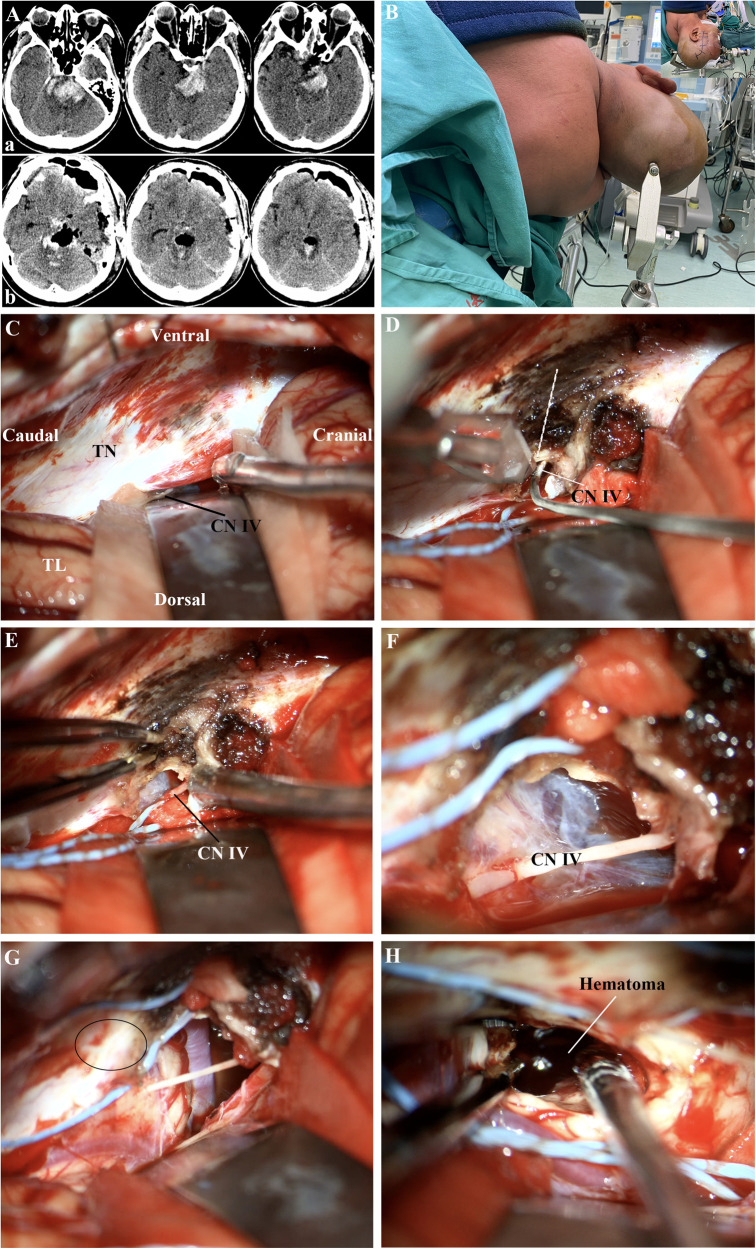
Left subtemporal trans-tentorial approach for pontine hematoma evacuation. (A) Cranial CT scans reveal pontine hemorrhage (a) and postoperative follow-up imaging (b) (B) The patient was placed in the left lateral position with the zygomatic arch maintained horizontal; the head was flexed downward at approximately 20°, and a preauricular curved incision was made (Upper right inset). (C) The arachnoid membrane at the temporal base was dissected to release cerebrospinal fluid, and gravity-assisted retraction of the temporal lobe was performed to expose the tentorium cerebelli and trochlear nerve. (D,E) The tentorium cerebelli was incised posterior to the trochlear nerve (Dashed line), with electrocoagulation applied intraoperatively during the incision. (F) After tentorial incision, the trochlear nerve was confirmed to be completely preserved, and the pons was fully visualized. (G,H) The surgical access was achieved via the supratrigeminal zone (Solid black circle) (G), followed by thorough hematoma evacuation (H) TC, Tentorium cerebelli; CN Ⅳ, Trochlear nerve.
